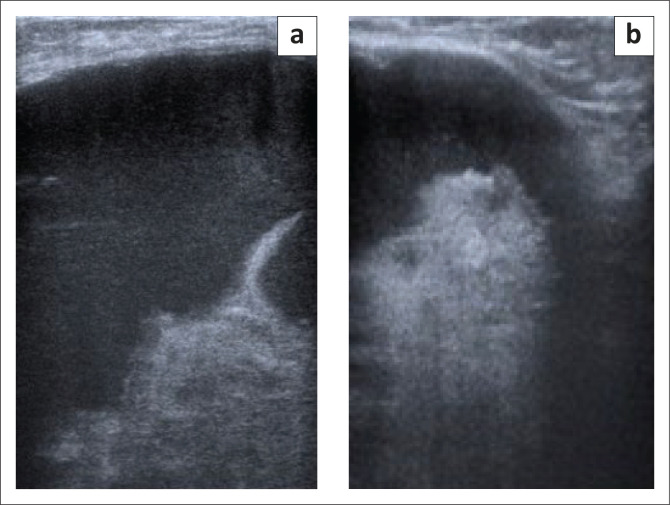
Axial/transverse view (a) and sagittal/ longitudinal view (b) of the left breast on initial ultrasound, demonstrating a large anechoic mass with multiple septations and irregular walls with debris.
